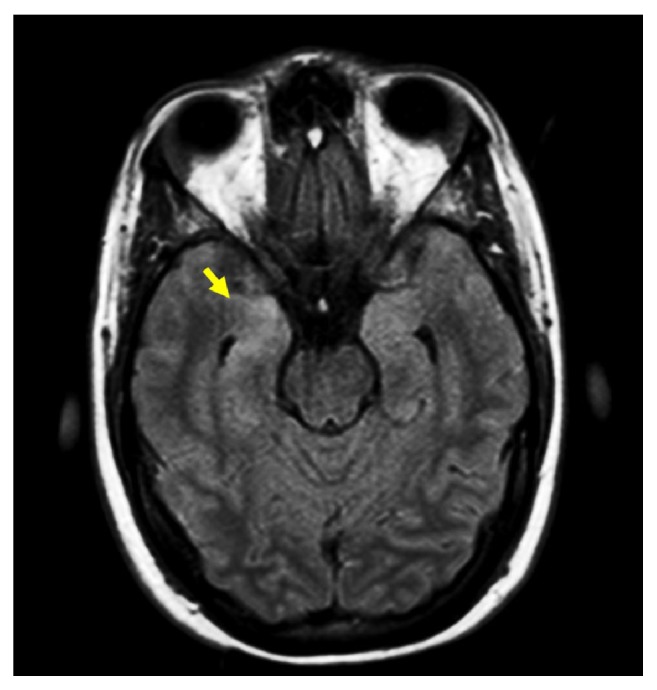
Brain MRI of patient with positive Anti-NMDAR encephalitis*. Arrow shows cortical hyperintensity in uncus right and hippocampus with T2-FLAIR technique.
